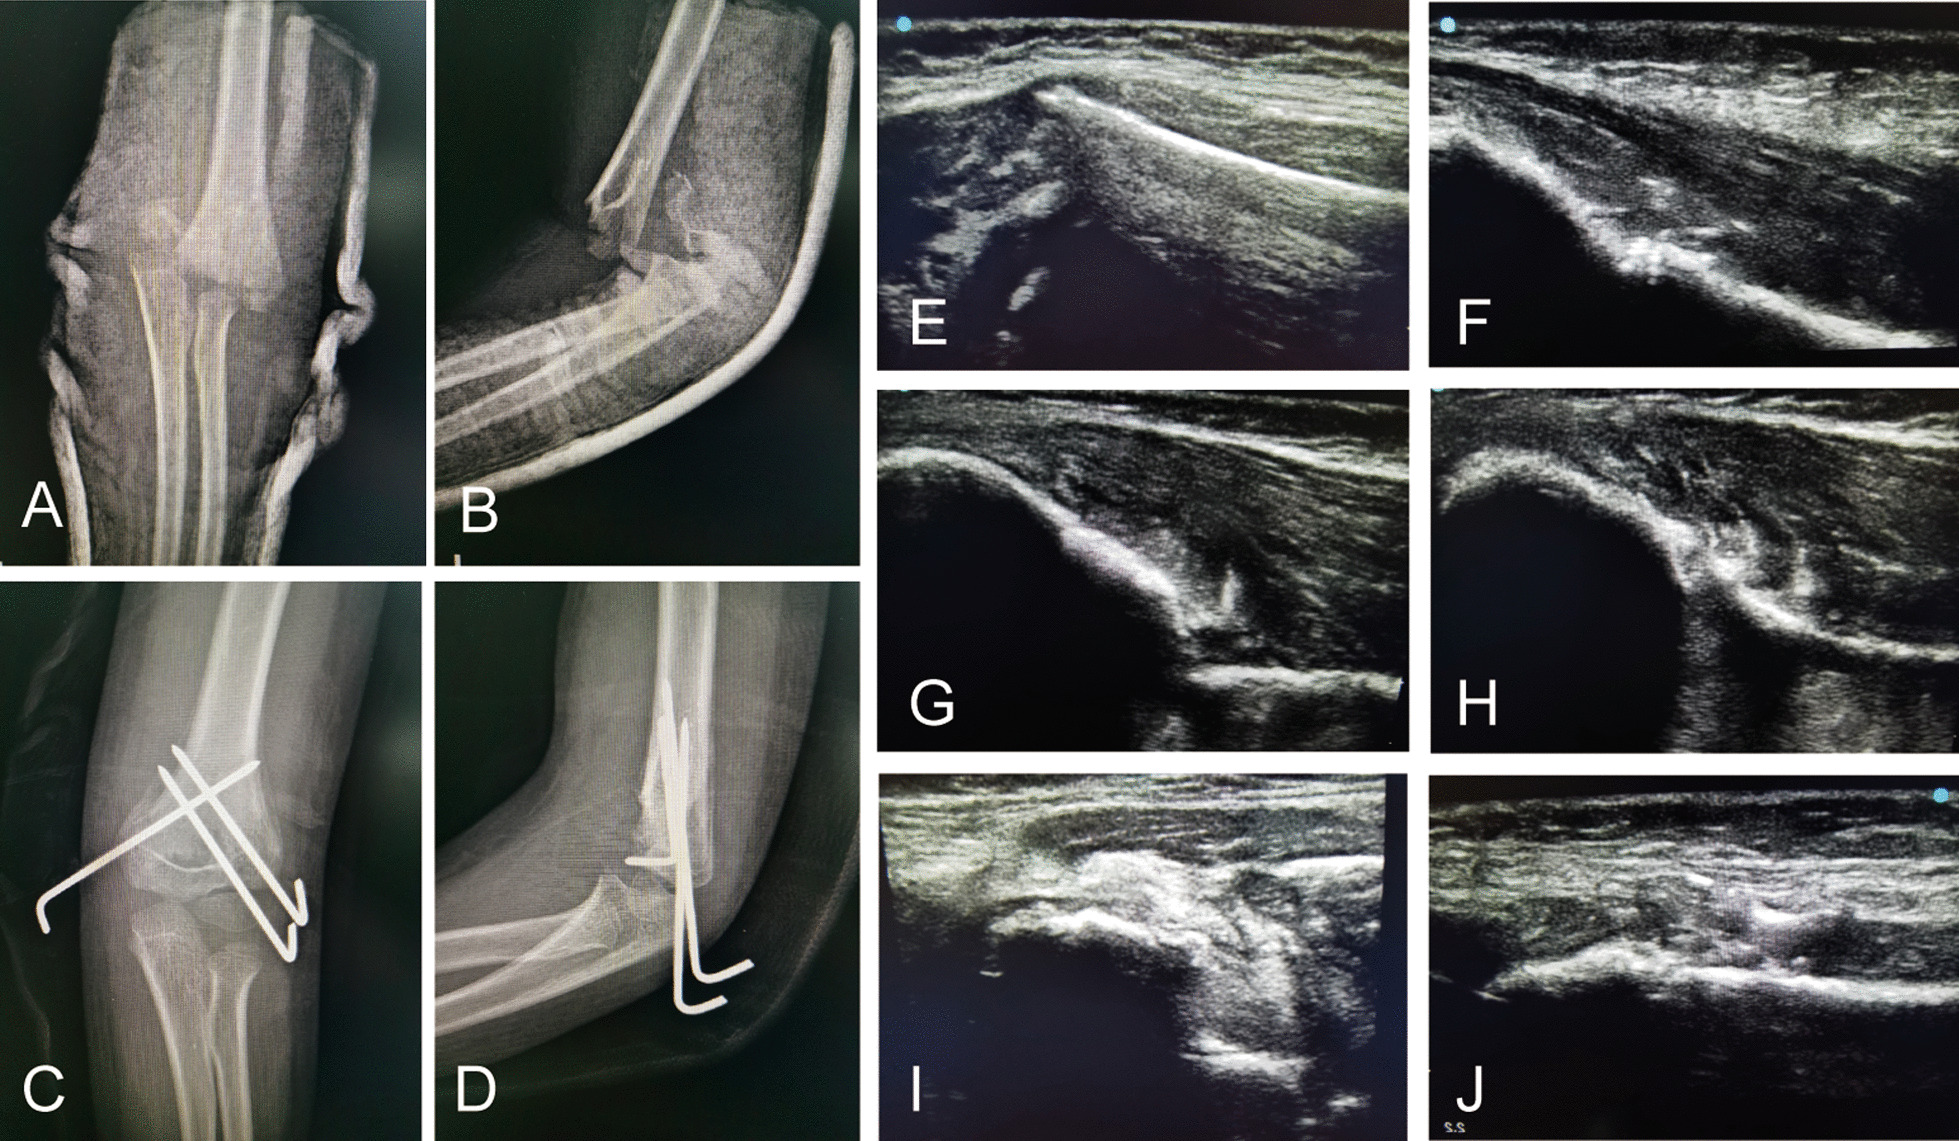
a Preoperative anteroposterior radiogram of the patient with Gartland III humeral fracture; b Preoperative lateral radiogram. c Postoperative anteroposterior radiogram after CRPP; d Postoperative lateral radiogram after CRPP. e Lateral, g medial and i posterior sonogram of forearm shows softtissue swelling around elbow and medial displacement of distal humerus; f Lateral, h medial and j posterior sonogram after reduction shows distal humerus back in its normal position
